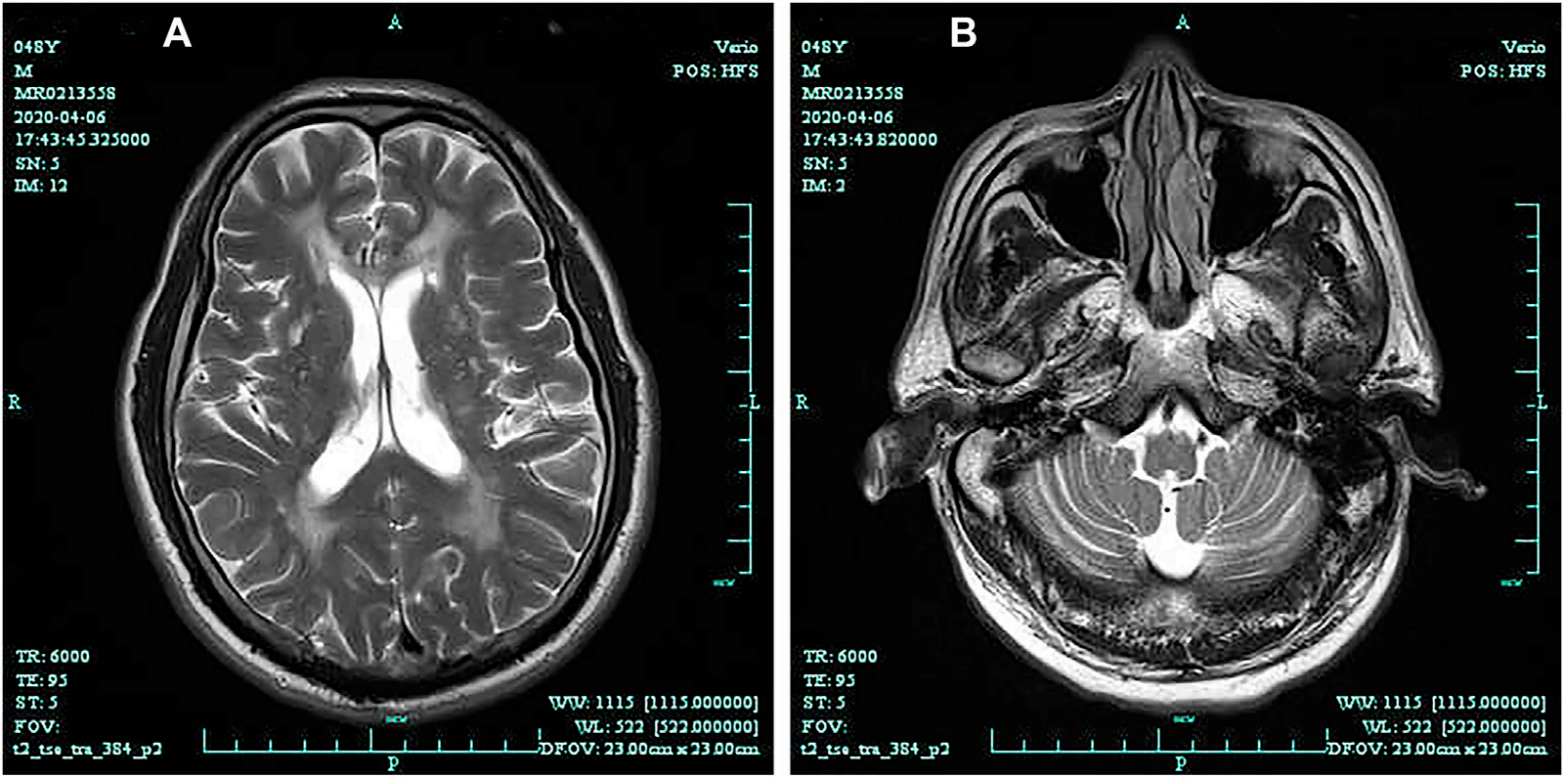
Brain magnetic resonance imaging (T2-weighted) shows diffuse leukoencephalopathy (A); Brain magnetic resonance (T2-weighted) shows cerebellar atrophy (B).
